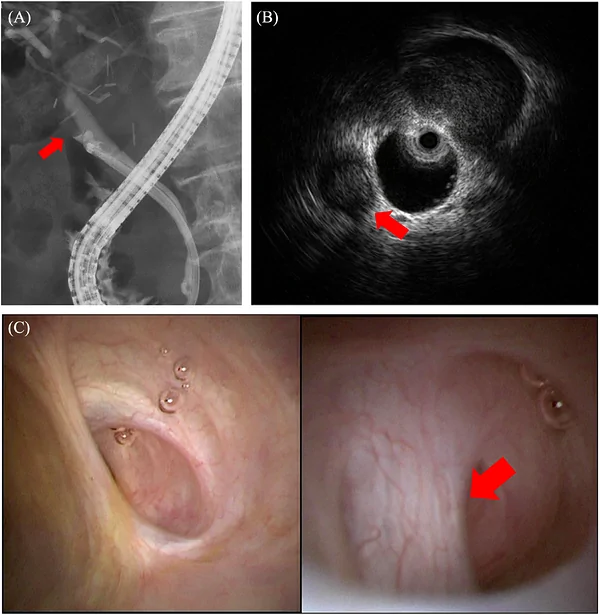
Endoscopic findings. (A) Cholangiography demonstrated an eccentric filling defect at the remnant cystic duct stump (red arrow). (B) Intraductal ultrasonography (IDUS) demonstrated a well‐defined hypoechoic lesion within the remnant cystic duct without disruption of the adjacent bile duct wall (red arrow). (C) Peroral cholangioscopy showed a smooth mucosal surface with a smooth submucosal‐like elevation at the remnant cystic duct stump (red arrow).
